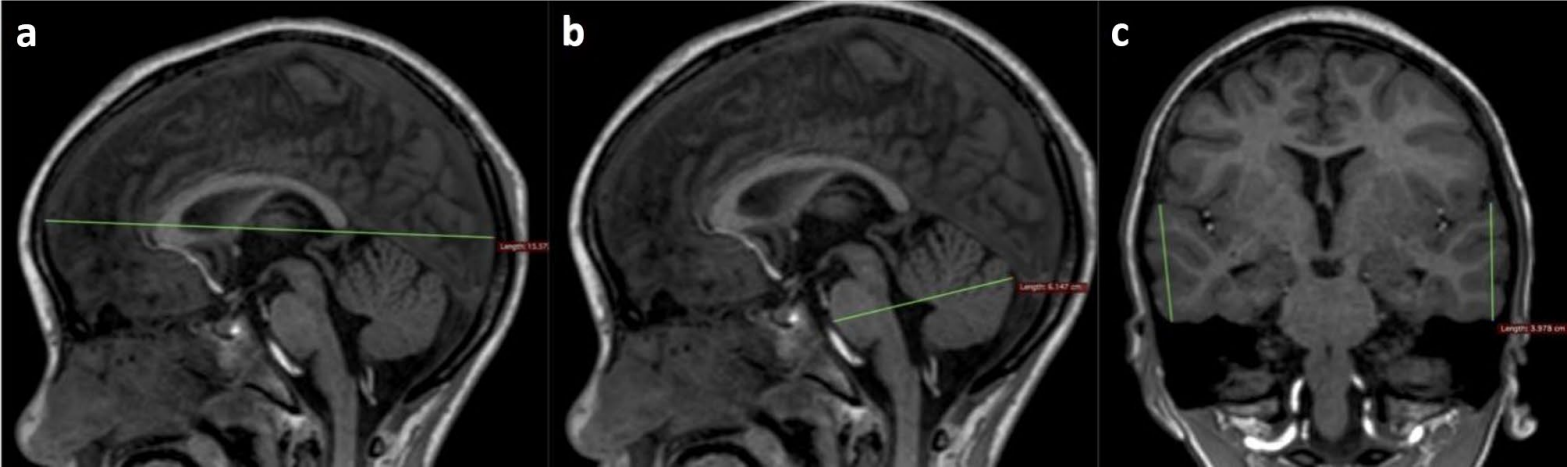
Linear measures on T1W MR images. Fronto-occipital measure on the midline sagittal image (a). Pons cerebellar thickness on midline sagittal image (b). Temporal height in the coronal plane (c)
